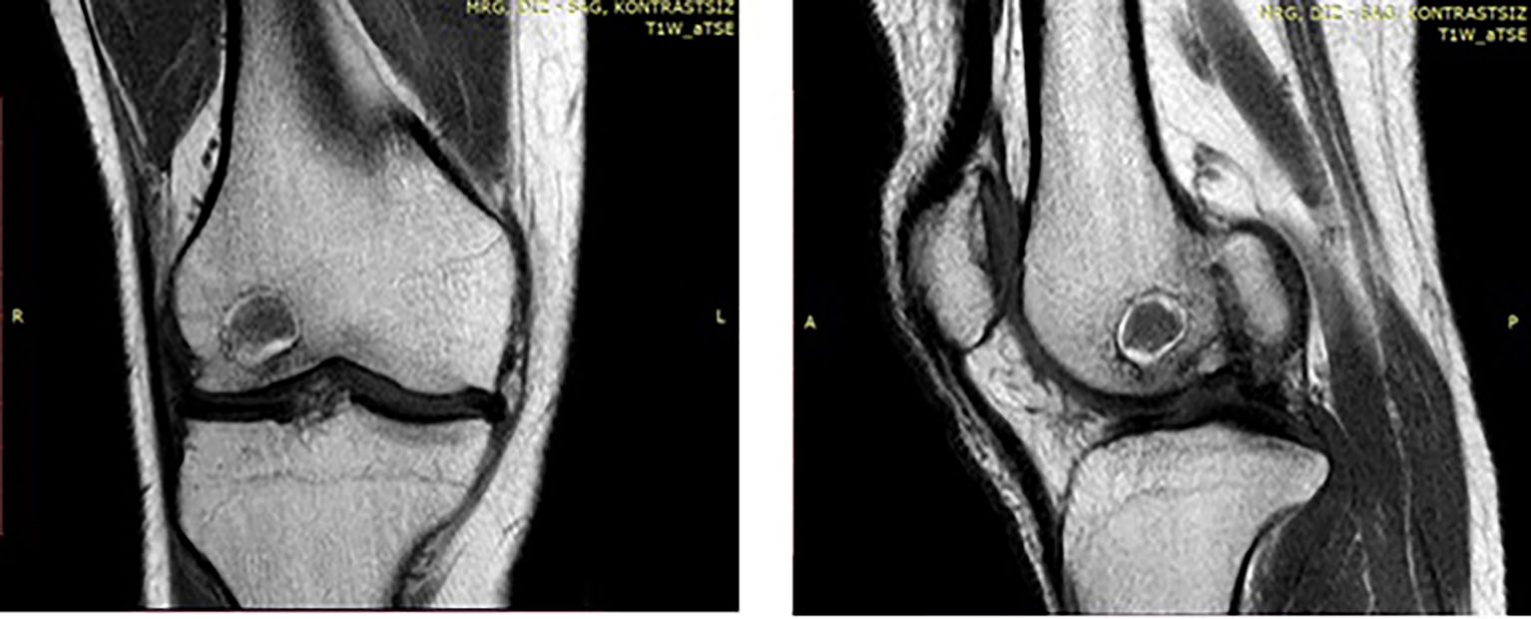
Penumbra sign in the MRI imaging of the patient.
